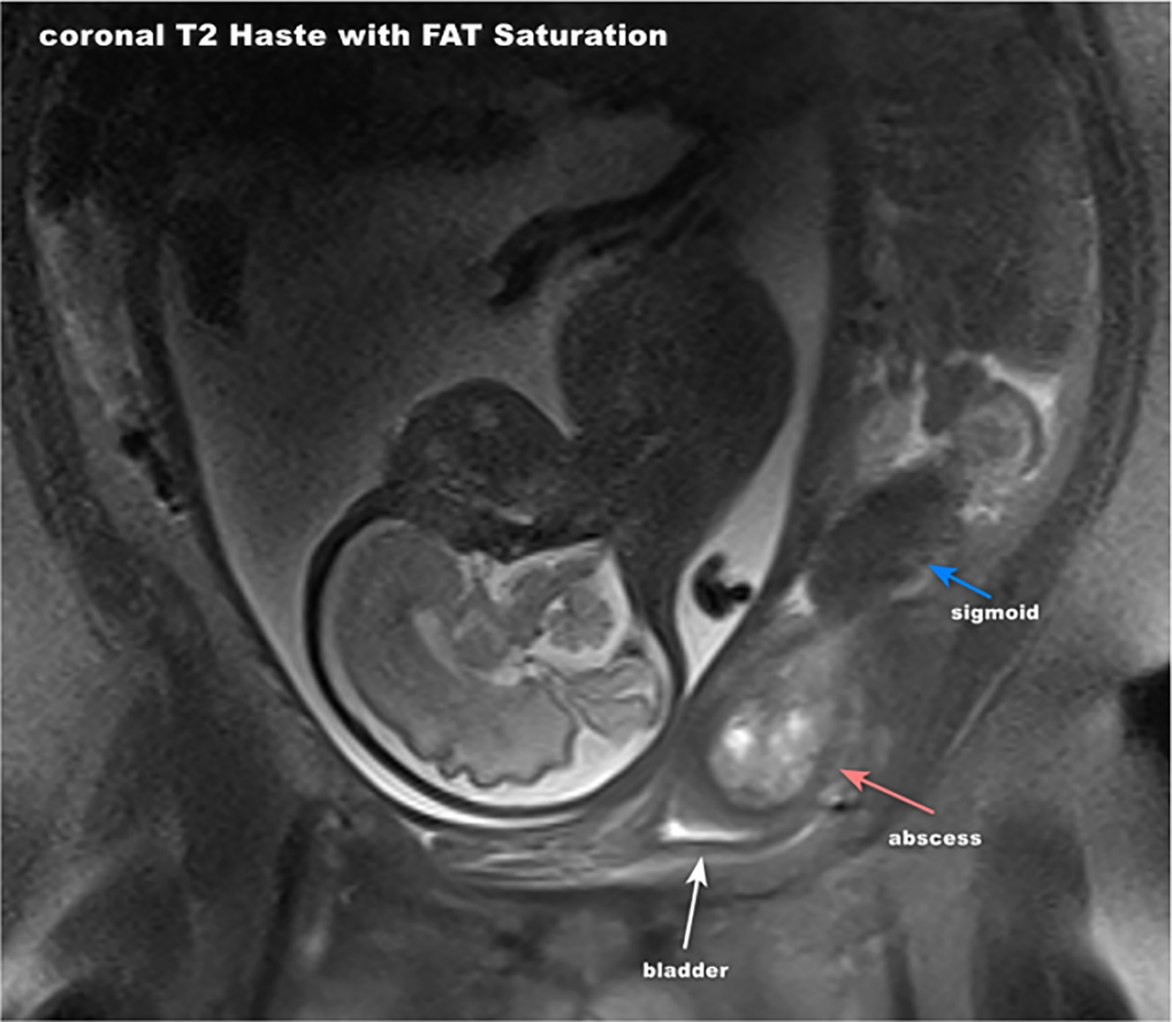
A 6 × 3 × 4.5 cm edematous cystic teardrop shaped structure abutting the sigmoid bowel with surrounding edema visualized on MRI abdomen/pelvis.
